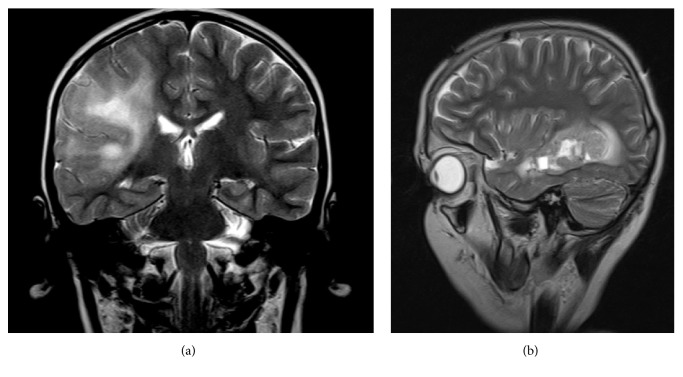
Magnetic resonance imaging of chosen gliomas. (a) Contrast-enhancing heterogenous diffuse infiltrating tumor involving two lobes with diffuse edema (P4, coronary, postcontrast T2), (b) recurrent well-demarcated glioblastoma surrounded with irregular edema (P5, sagittal, postcontrast T2).
